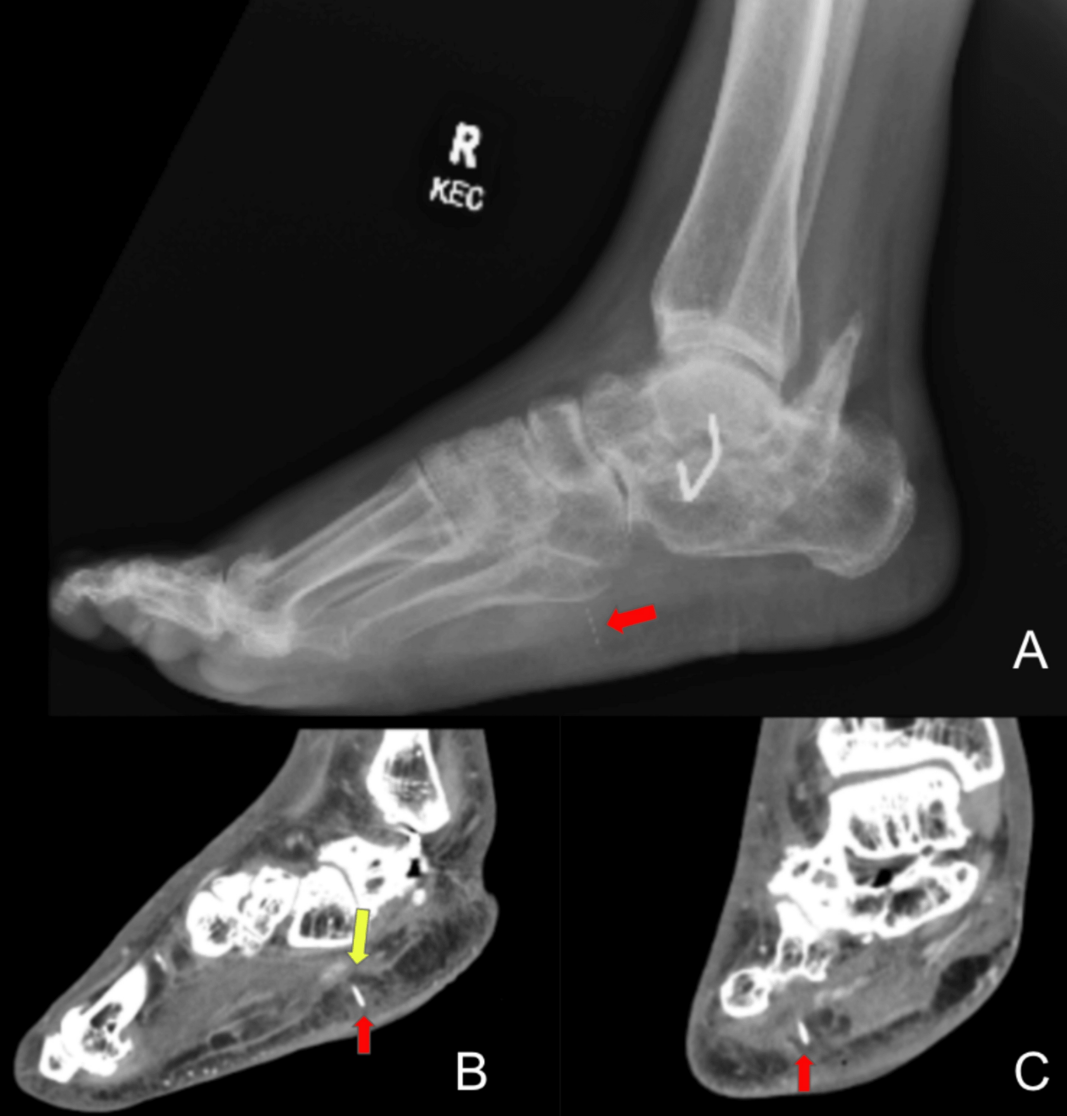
Lateral X-ray of the right foot (A), sagittal (B) and coronal (C) CT soft tissue window before wound debridement on first admission. A small foreign body, later found to be an insulin needle, measuring 1.3 cm in length, was noted on the plantar surface, 1 cm deep into the skin surface (red arrow). The surrounding area of the foreign body demonstrated soft tissue swelling and ectopic air (yellow arrow), suggestive of abscess formation.
